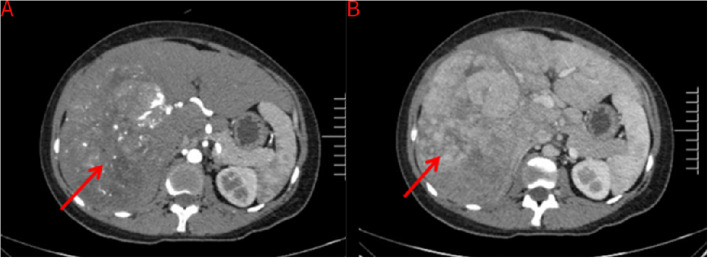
A Contrast enhanced CT revealed the mass had heterogeneous enhancement in the arterial phase. B Contrast-enhanced CT scan demonstrated no marked change in intensification in the venous phases
